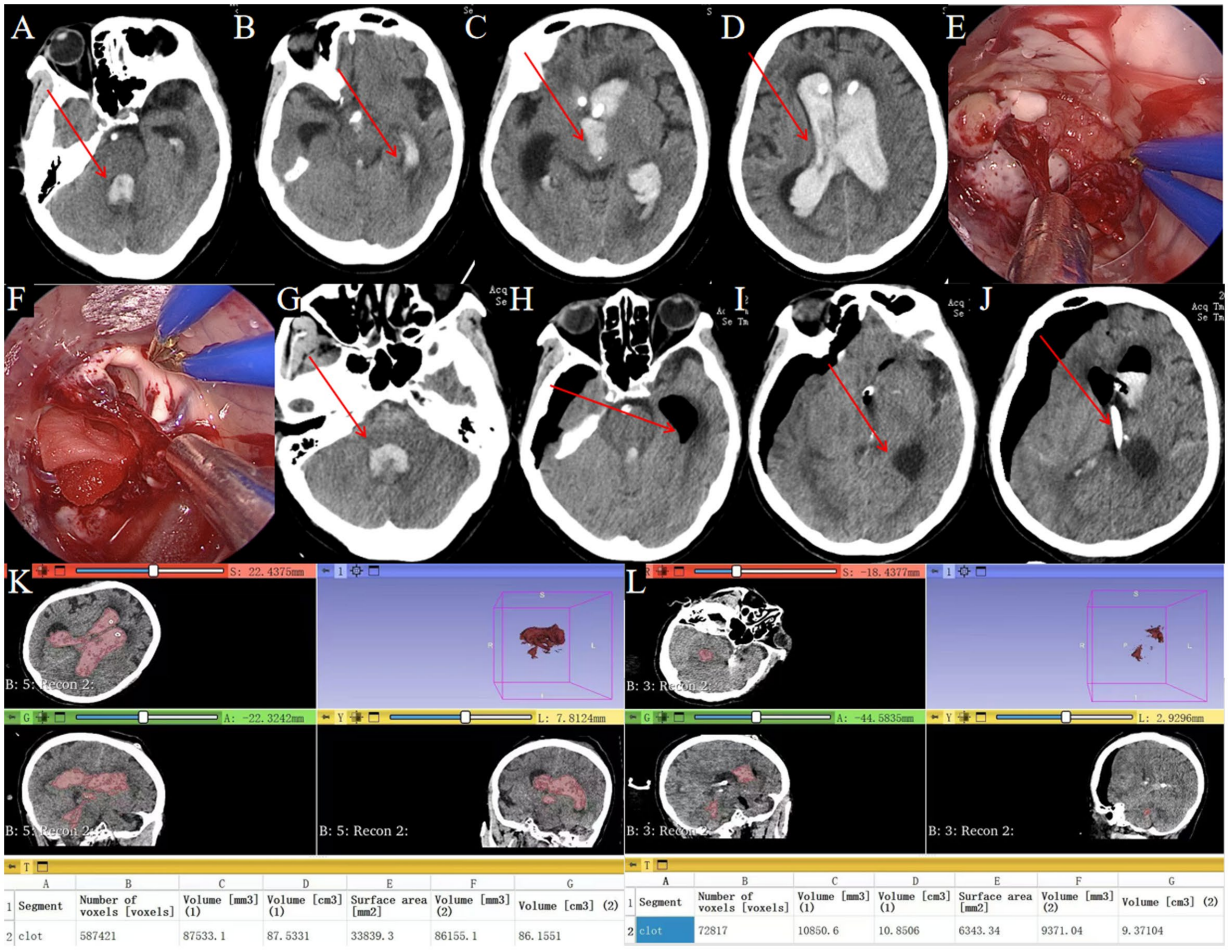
Endoscopic removal of hematoma in complete ventricular casting. (A–D) Preoperative head CT showing complete ventricular casting (→ arrows mark hemorrhage in the fourth, third and lateral ventricles). (E) Intraoperative neuroendoscopic view of hematoma evacuation from the lateral ventricle using a transparent sheath. (F) Intraoperative neuroendoscopic view confirming clearance of the lateral ventricle. (G) Postoperative CT showing residual hematoma in the fourth ventricle (→ arrow highlights residual clot). (H,I) Postoperative CT demonstrating hematoma removal in the lateral and third ventricles (→ arrows denote evacuated regions). (J) Postoperative CT showing drainage tube to drain residual hematoma (→ arrows denote drainage tube). (K) Preoperative hematoma volume: 87.5 mL. (L) Postoperative hematoma volume: 10.8 mL.
